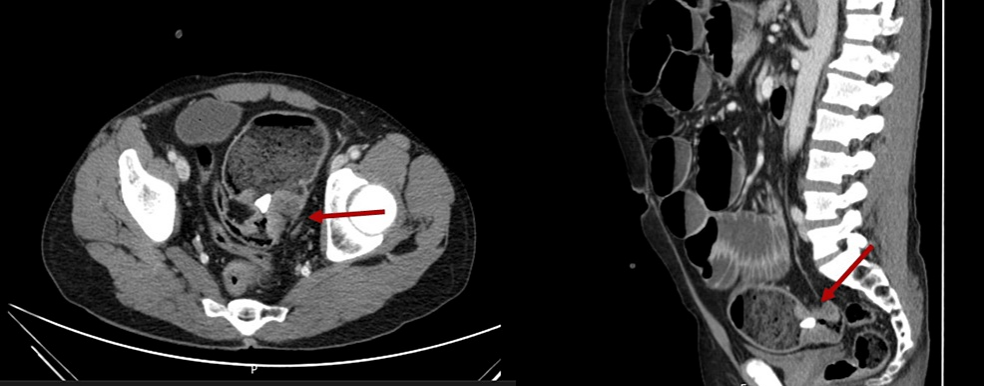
Abdominopelvic CT scan Findings of an apple core lesion situated at the rectosigmoid transition, containing a foreign body within, leading to complete colonic obstruction.
